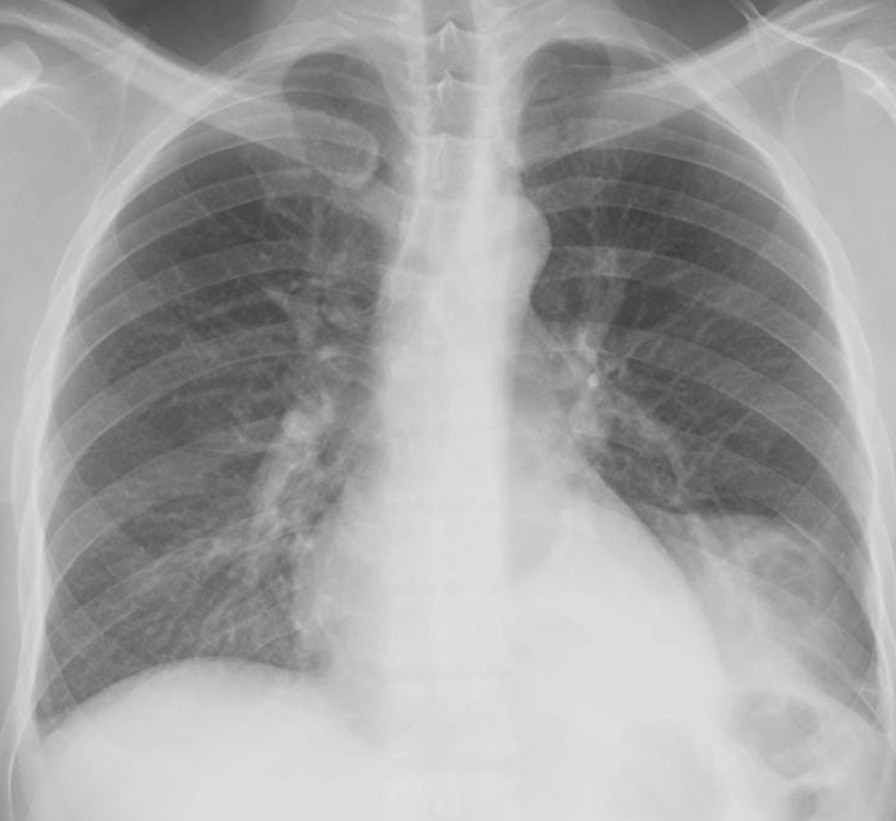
Chest X-ray performed on admission showing an infiltrative shadow in the left lower lung field
